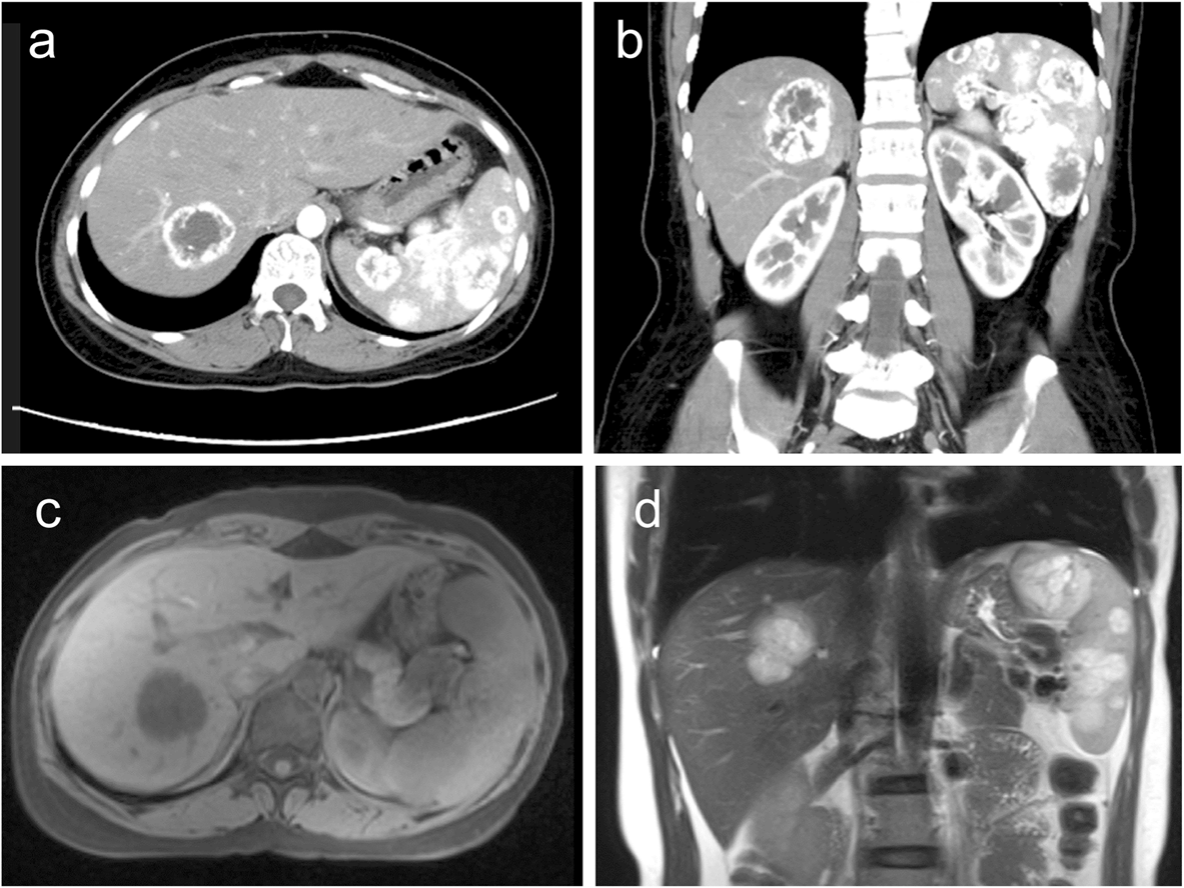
Preoperative images of computed tomography (CT) and magnetic resonance imaging (MRI) scans. Both the axial (a) and the coronal (b) CT images show multiple low-density nodules with enhanced nodule boundaries in the spleen and the liver. MR images of the splenic and hepatic masses show a low signal intensity on T1-weighted MR (c) images and a high signal intensity on T2-weighted MR (d) images.
